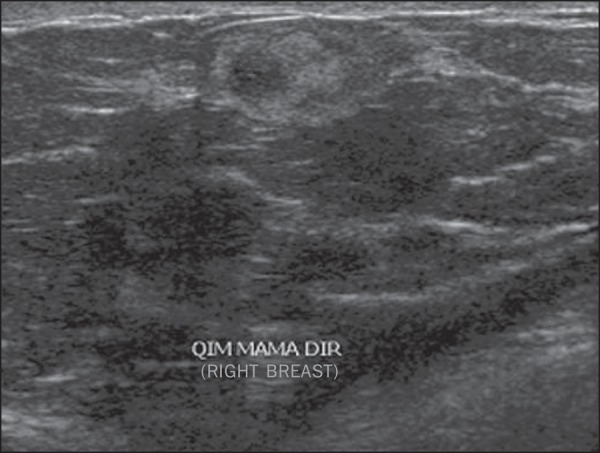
Metastasis from leiomyosarcoma*. Ultrasonography
